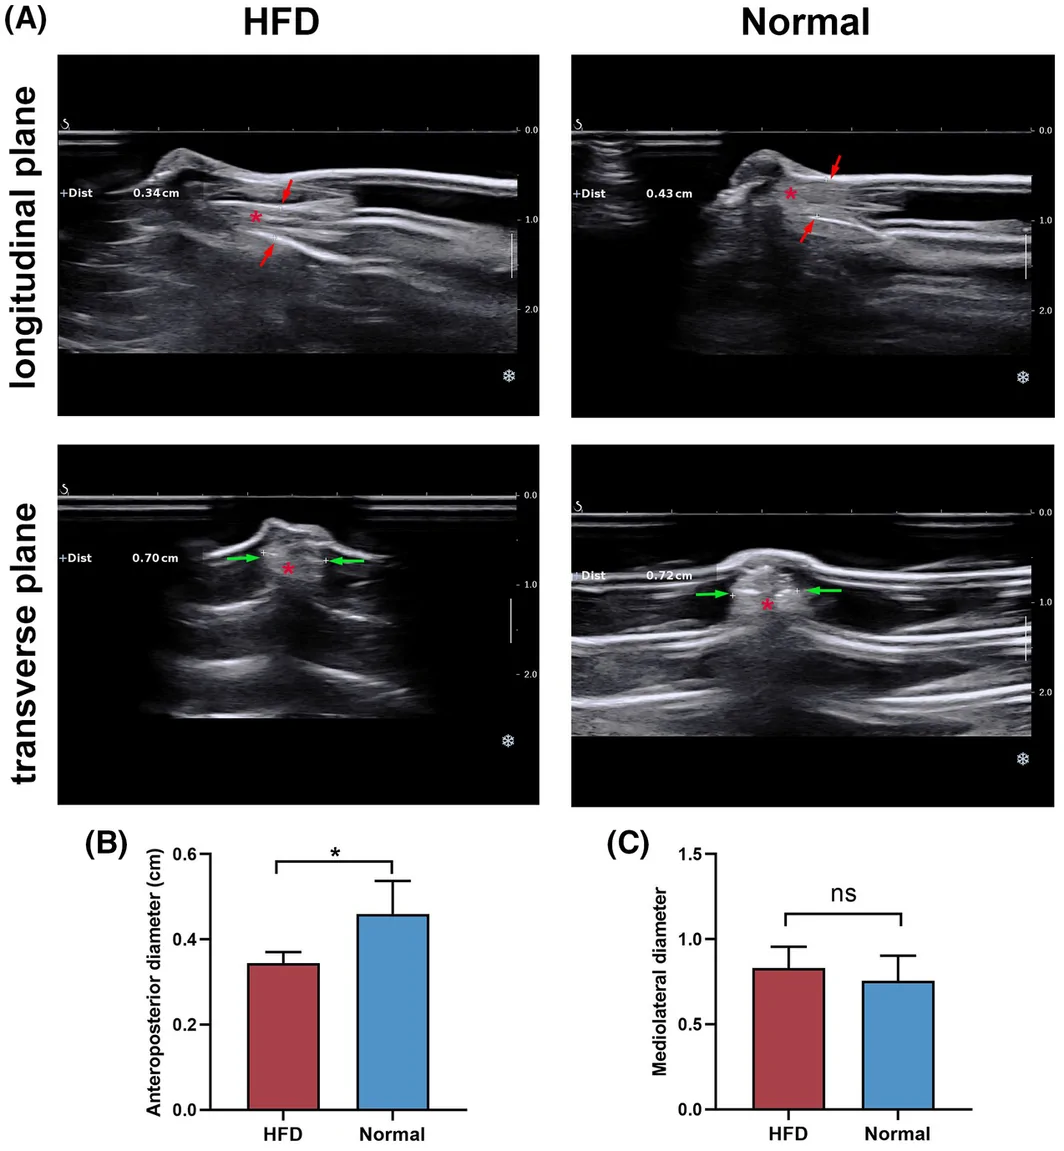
Ultrasound morphological assessment of the Achilles tendon (*n* = 4 per group). (A) Representative longitudinal and transverse ultrasound images of the Achilles tendon in the HFD and Normal groups. Red stars indicate the Achilles tendon; red arrows denote the anterior and posterior borders, while green arrows indicate the medial and lateral borders. The depth scale shown on the right side of each ultrasound image is in centimeters, and the vertical white bar indicates 0.5 cm. (B) The anteroposterior diameter (AP) of the Achilles tendon was measured as the distance between the anterior and posterior borders at the thickest region. (C) The mediolateral diameter (ML) was determined as the distance between the medial and lateral borders on transverse images. Data are presented as mean ± SD. Statistical comparisons were performed using an unpaired two‐tailed Student's *t*‐test. P* < 0.05, *P* < 0.01, P* < 0.001, and ns indicates no significant difference.
